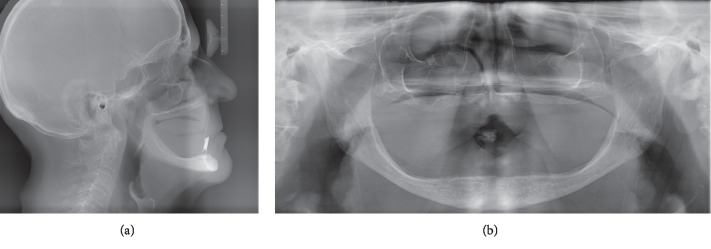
(a) Preoperative cephalometric radiograph. (b) Preoperative panoramic radiograph.
